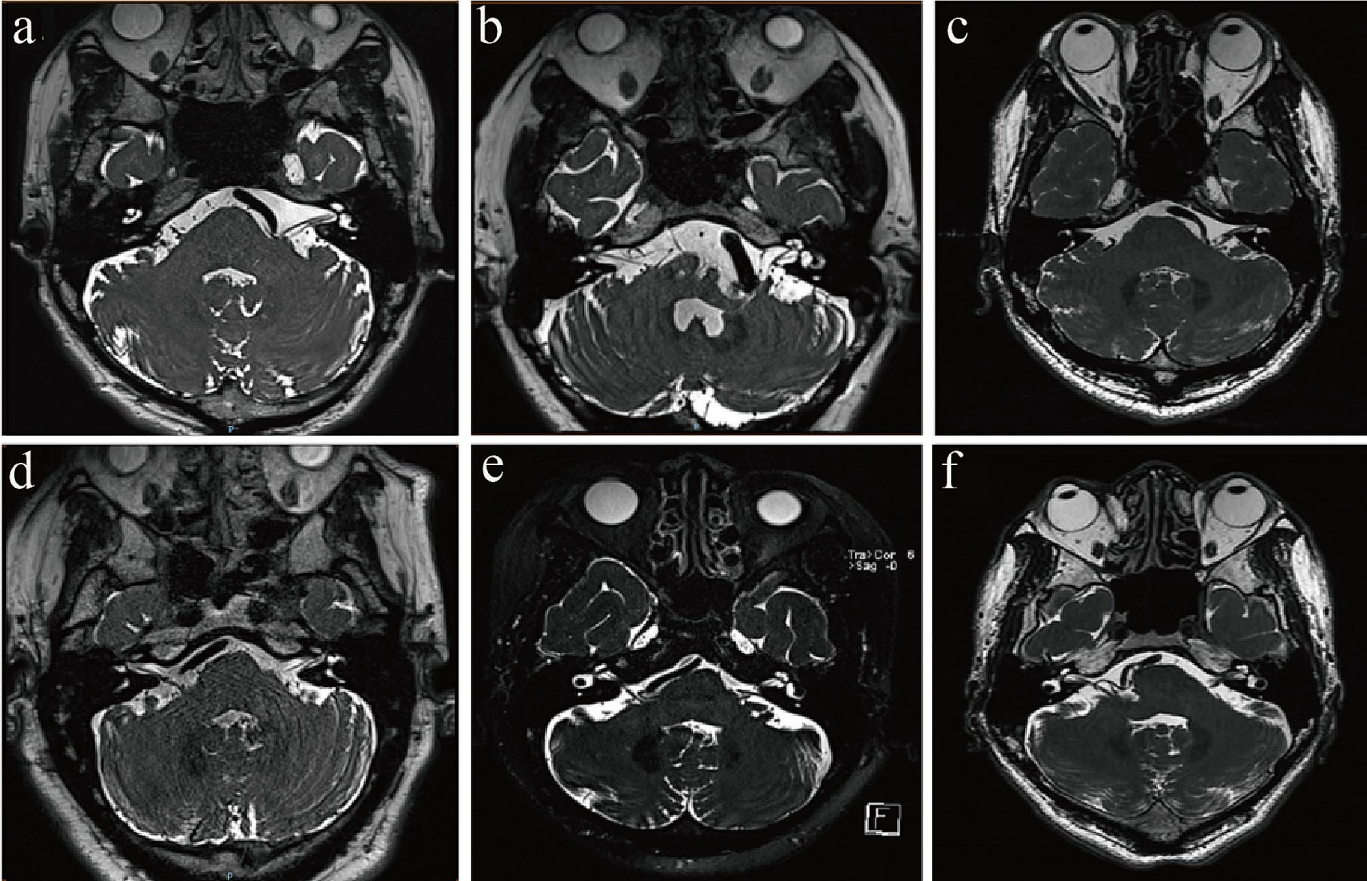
Preoperative MRI images of VA-involved patients. The images demonstrated that the REZ of the facial nerve was compressed by the VA. Panels a-c show left-sided HFS, while panels d-f depict right-sided HFS
